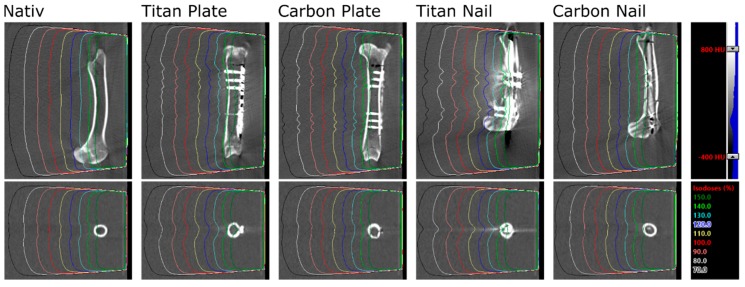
CT images and calculated dose distributions for all bones and implants at 5 cm immersion depth. Densities from −400 to 800 HU are shown in grey scale; isodose levels of a 6 MV, 20 × 20 cm^2^ field are shown in color.
